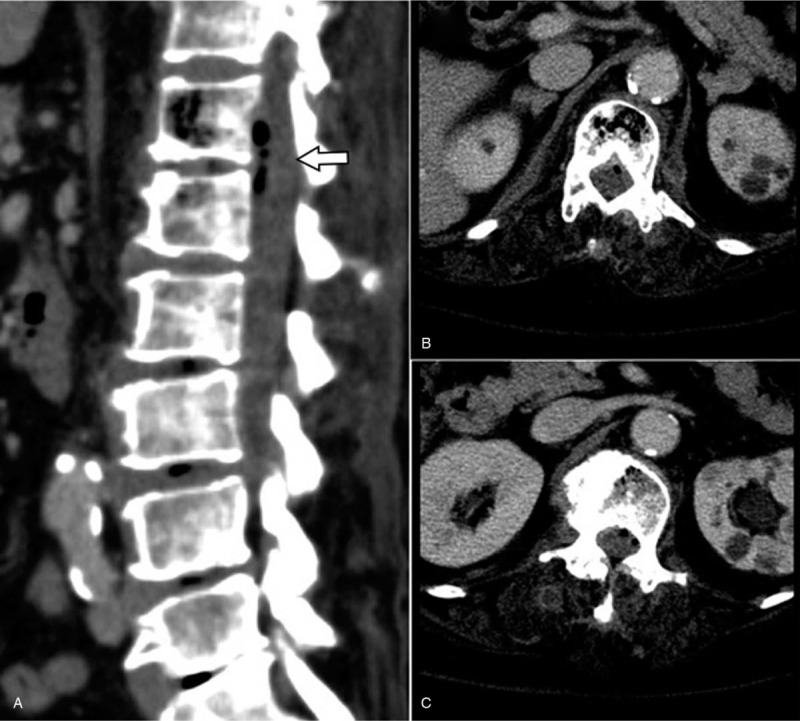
Follow-up computed tomography scan showing progressed emphysematous osteomyelitis at vertebral bodies of T12 (B) and L1, and newly developed air density at epidural space of T12-L1. (arrow) (A).
